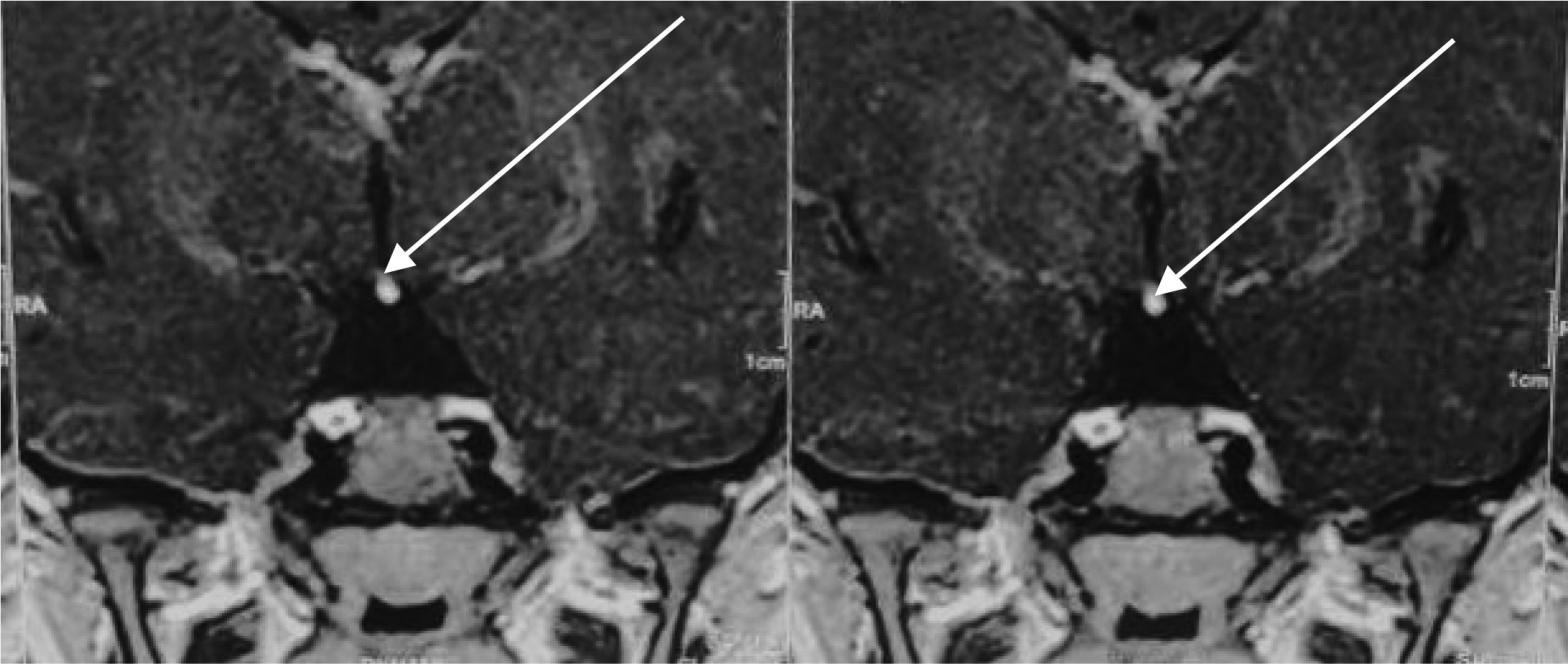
Coronal post‐contrast magnetic resonance imaging of the brain shows an enhancing nodule at the inferior aspect of the optic chiasma (white arrow), suggestive of an ectopic posterior pituitary gland.
